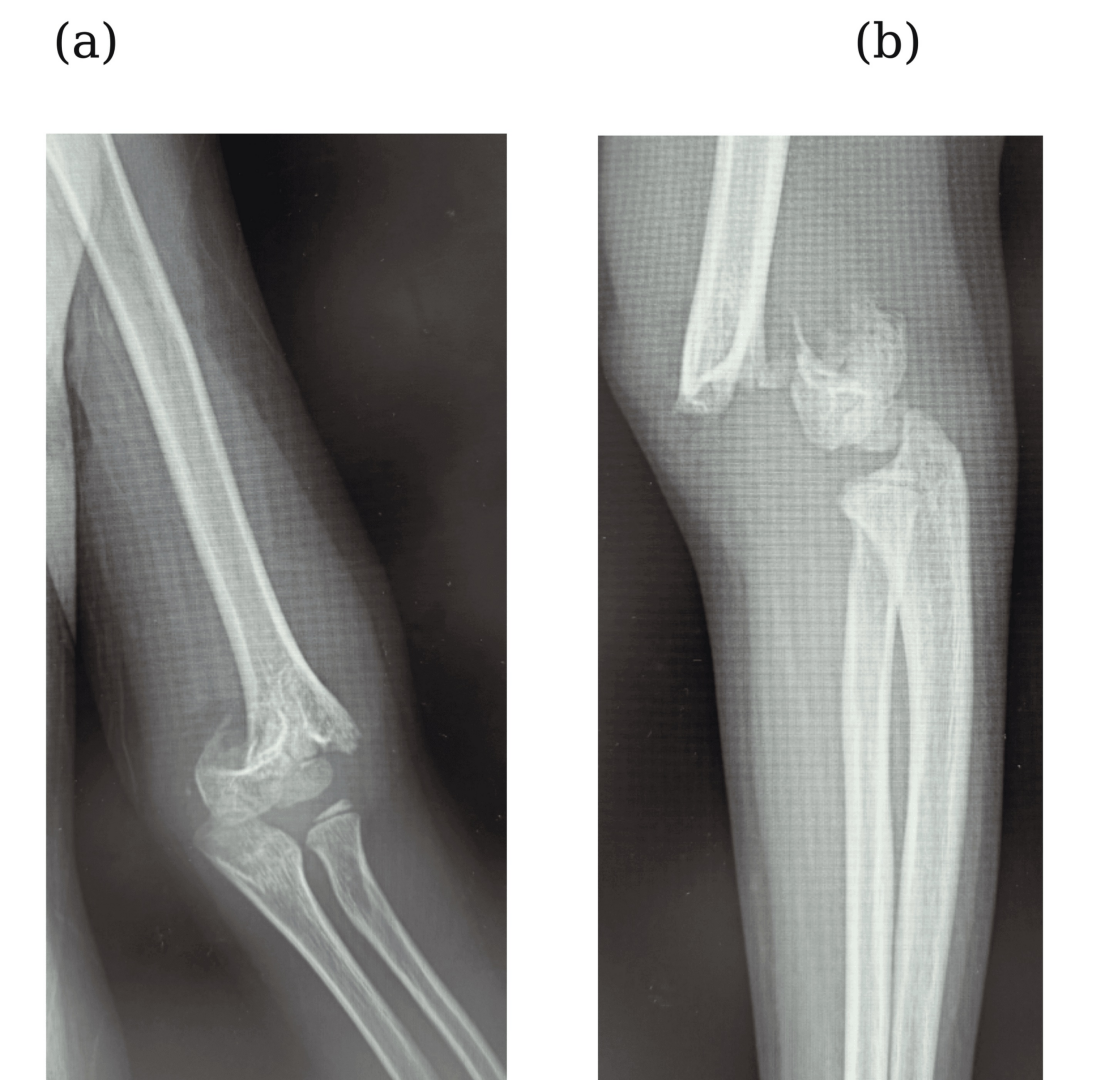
Initial plain radiograph in (a) frontal view and (b) lateral view taken at the first hospital visit.
